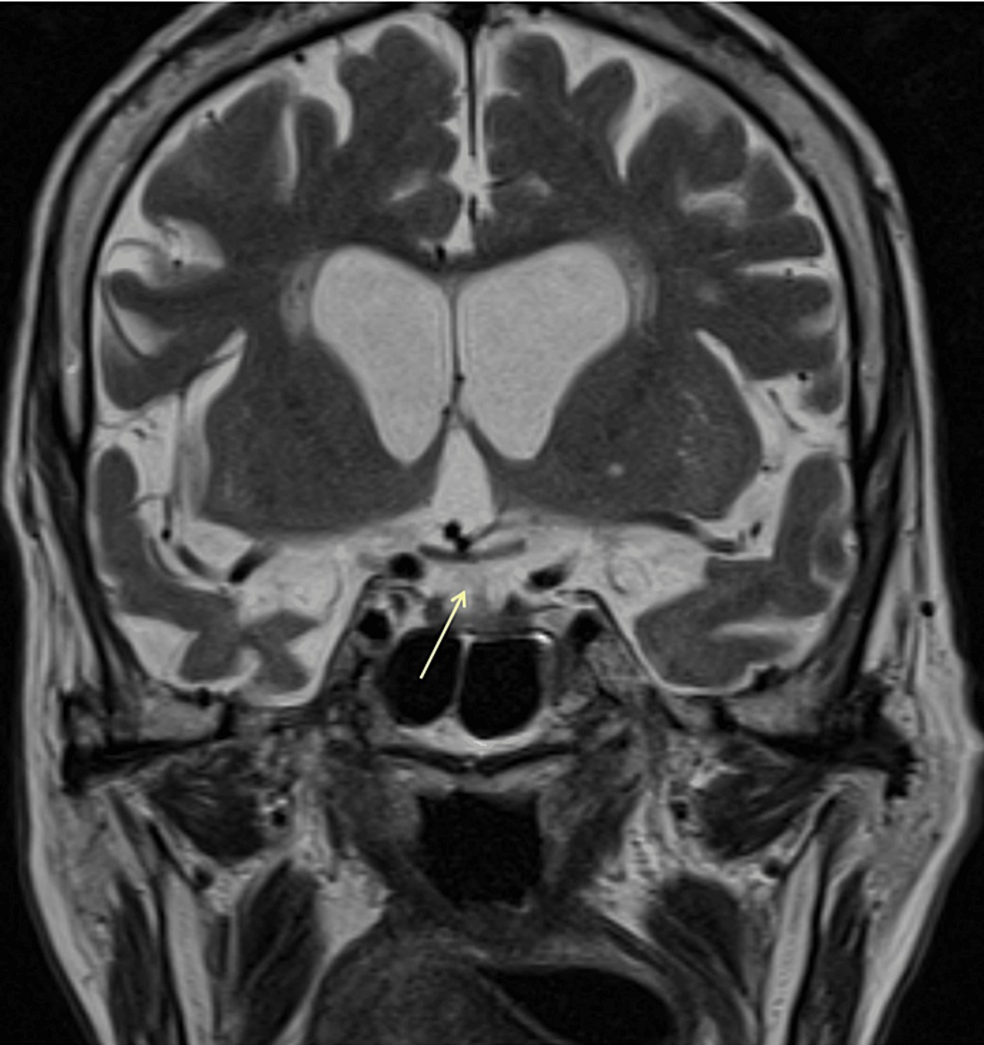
Right pituitary microadenoma on MRI. MRI - magnetic resonance imaging.
